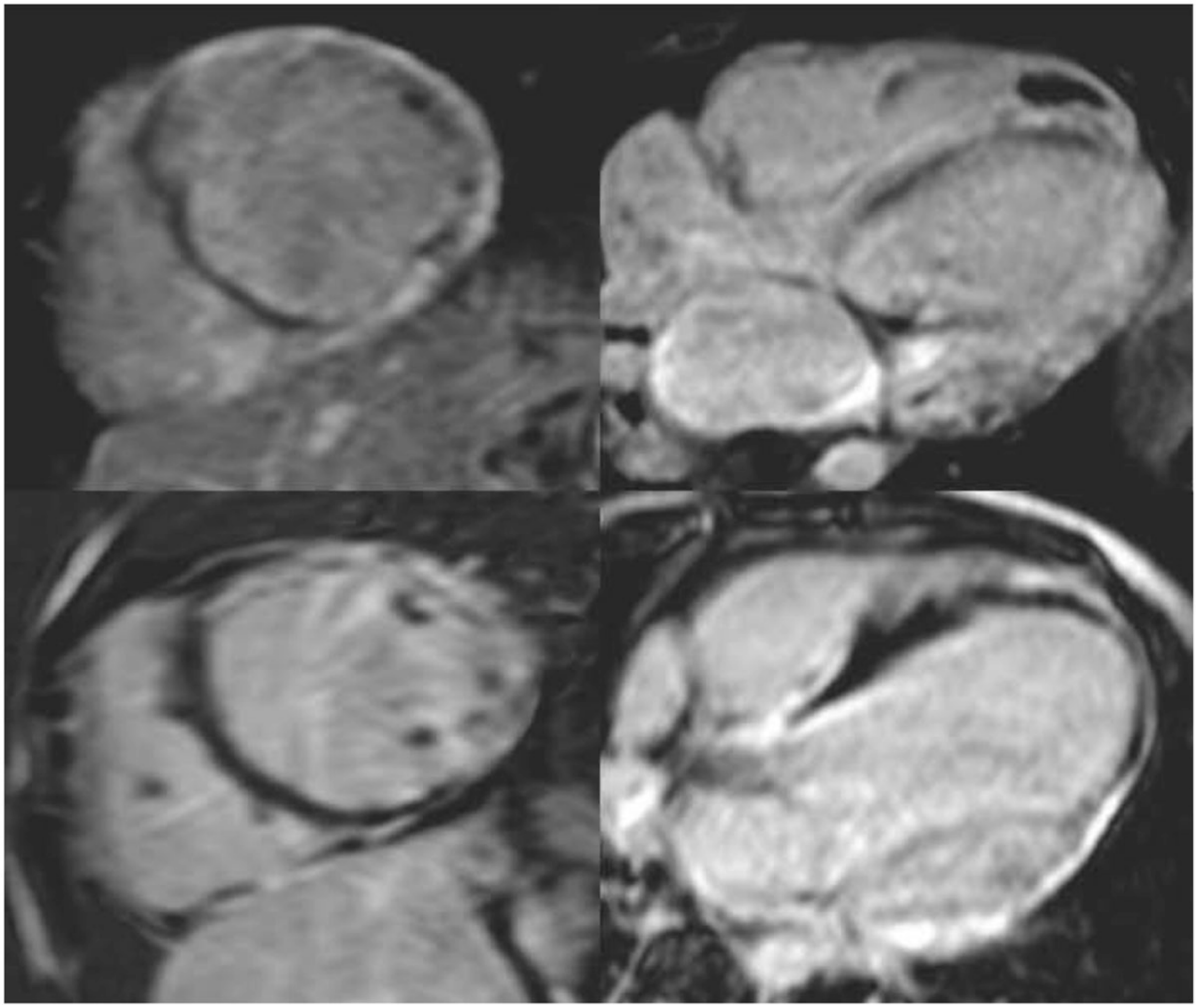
Cardiac MRI late Gadolinium enhancement, short axis view (right column) and four chamber views (left column) showing diffuse epi-cardial and transmural involvement in two different cases of myocarditis. Upper row are images of same case and lower row are of the second case.
